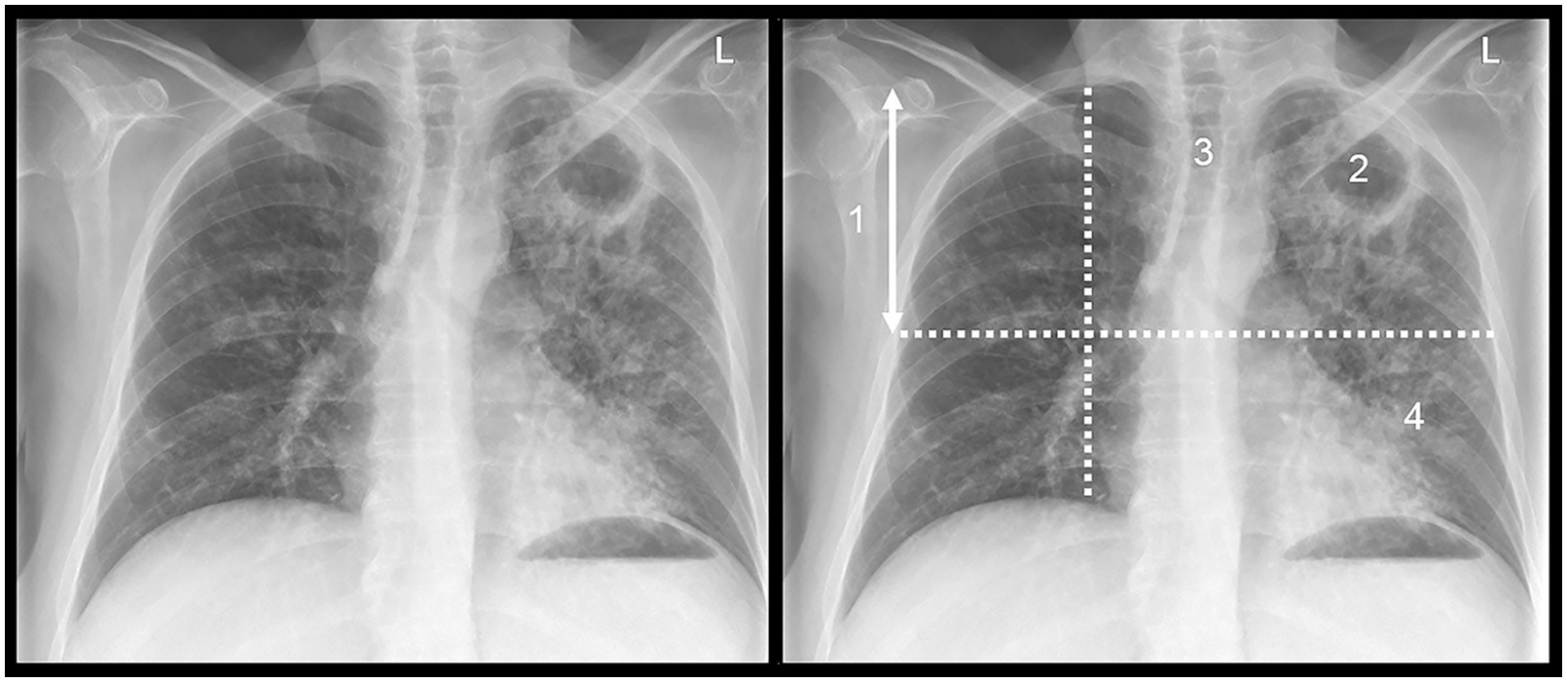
A posterior-anterior chest radiograph in a patient with typical adult-type smear-positive pulmonary tuberculosis. The major features are: (1) upper lung zone distribution; (2) cavitation; (3) volume loss; (4) acinar shadows.
